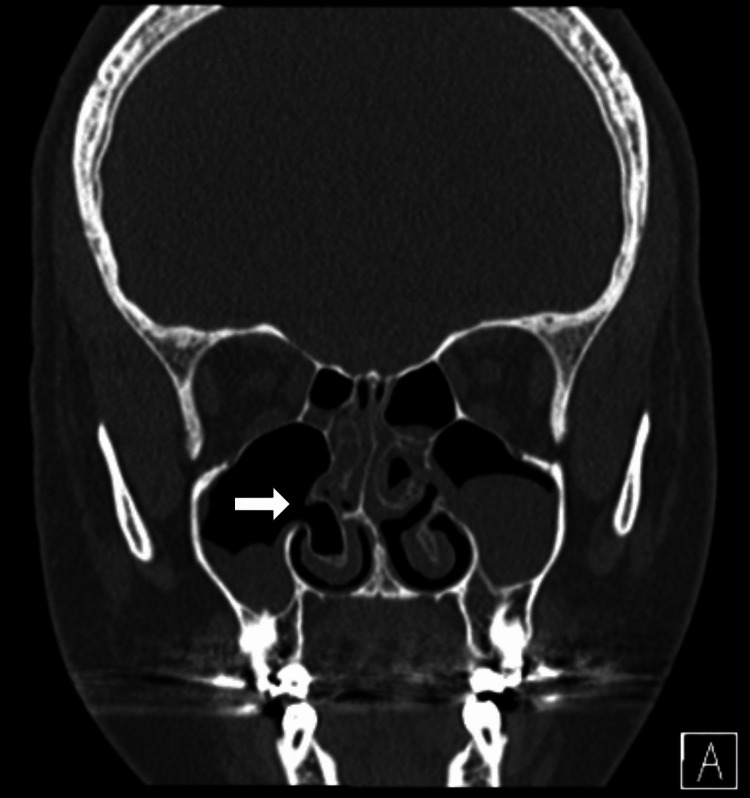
Non-contrasted paranasal sinuses CT scan, coronal view for case 1. Nasal findings include a pneumatized right-side inferior turbinate with a large opening into the ipsilateral maxillary sinus (arrow). Note that the inferior turbinate pneumatization pattern is communicating and show a mix between lamellar and bulbous types.
